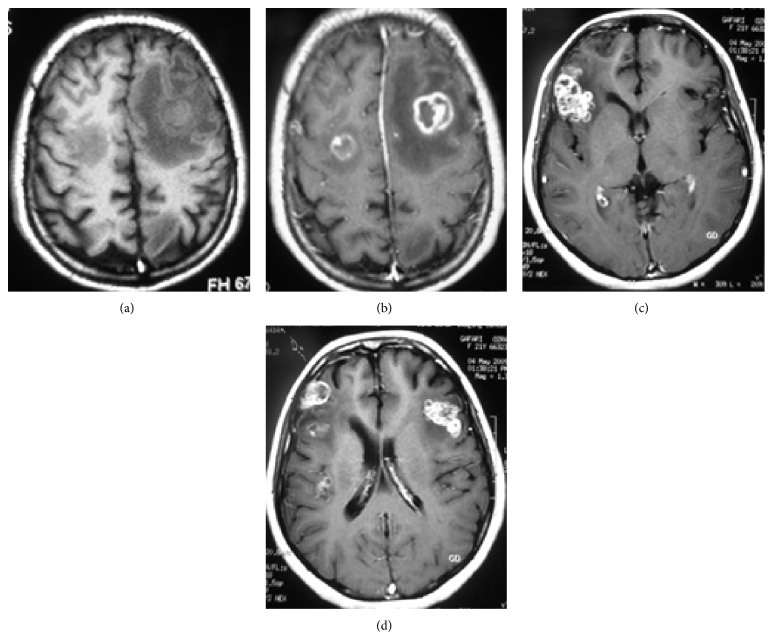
Tuberculous brain abscess. Axial pre- and postcontrast T1-weighted MR images ((a) and (b)) in a 38-year-old male with cognitive and speech disorders show two hypointense lesions in both frontal lobes with peripheral edema, which is severe on left side, and have thick ring-like enhancement (b). (c) and (d) Axial postcontrast T1-weighted MR images in a 22-year-old female with pulmonary miliary tuberculosis and 3-month history of headache, nausea, vomiting, and recent seizure demonstrate bifrontal irregularly enhancing lesions with mild peripheral edema.
